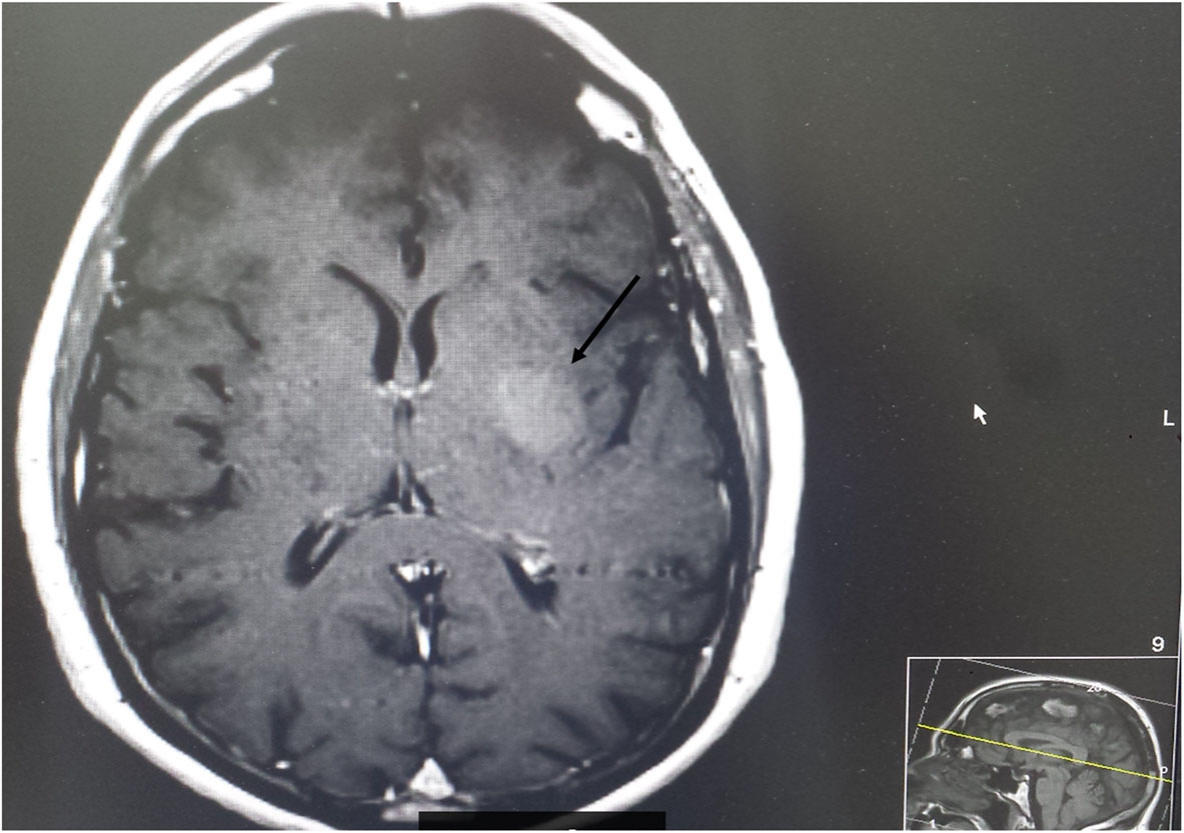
Magnetic resonance imaging scan. Arrow: tumor
